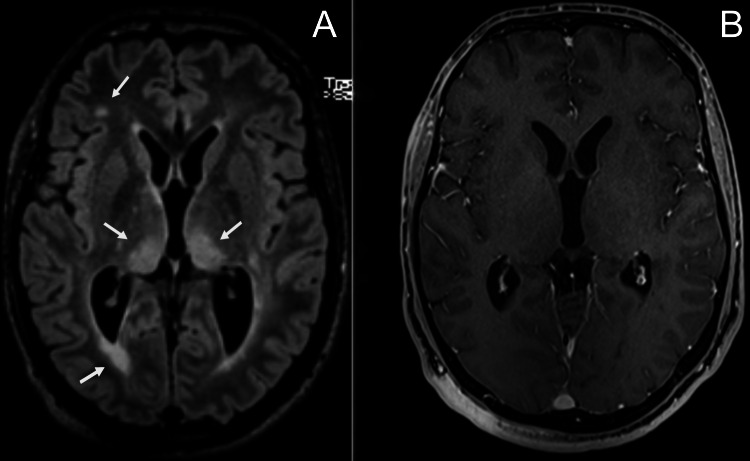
Brain magnetic resonance imaging, axial view. (A) Fluid-attenuated inversion recovery sequence (FLAIR) demonstrates several hyperintense lesions involving the deep white matter (mostly periventricular), the subcortical white matter in the right frontal lobe and both thalami (arrows); (B) they show no enhancement after administration of gadolinium on a fat-saturated T1-weighted volumetric interpolated breath-hold examination (VIBE) sequence. Their morphology and location are typical of focal areas of signal intensity (FASI) in the setting of neurofibromatosis type 1.
